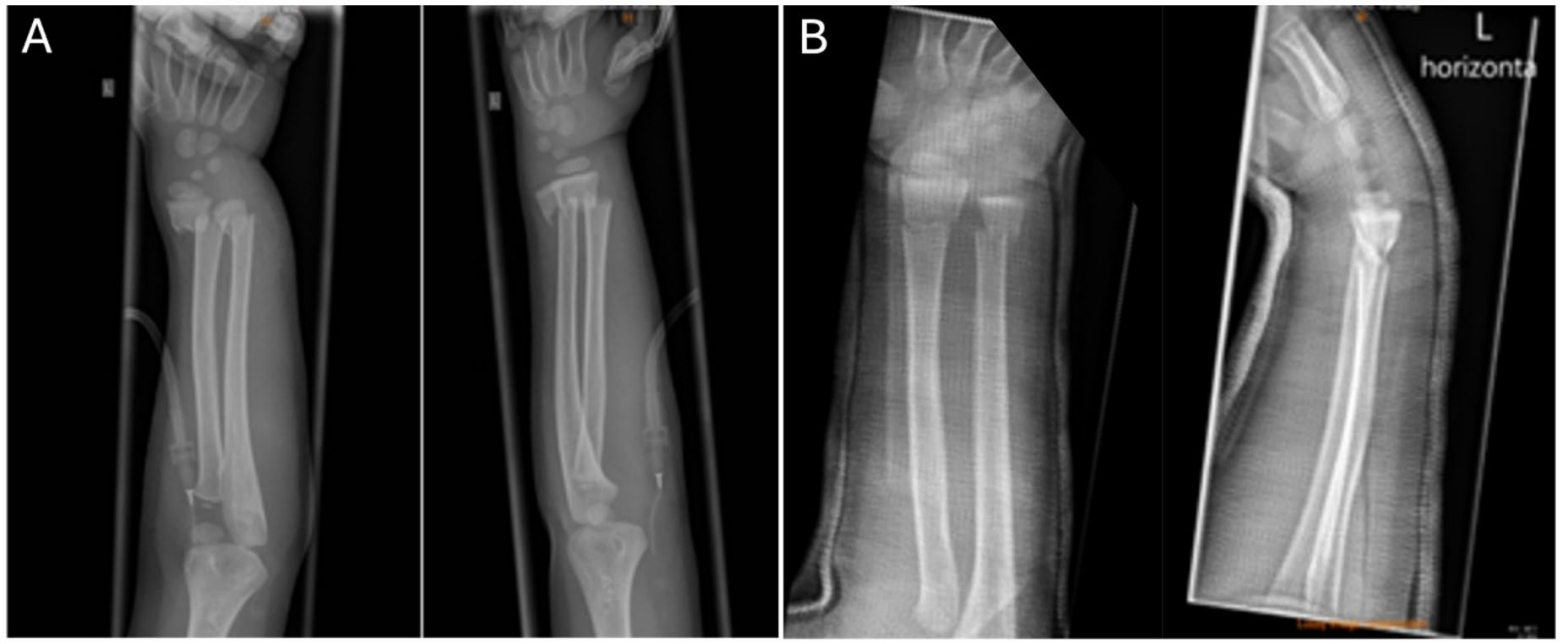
X-rays before (A) and after (B) closed reduction of the fully displaced forearm fracture.
